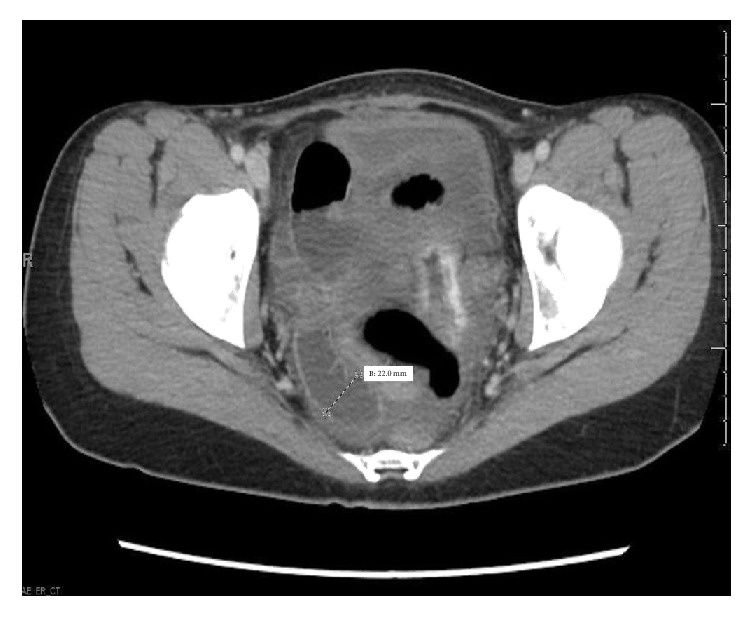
Axial CT image of the small bowel extending into the vagina. Normal thickness is less than 30 mm.
